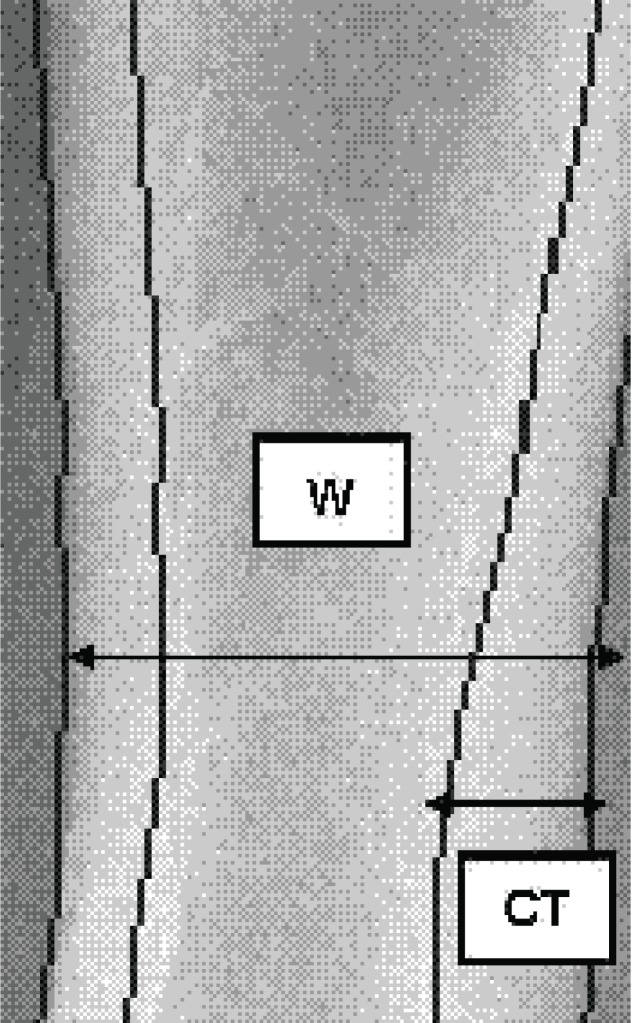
Principle for calculation of Metacarpal Index (MCI) by Digital X-ray Radiogrammetry. W, outer bone width of a metacarpal; CT, cortical thickness.
